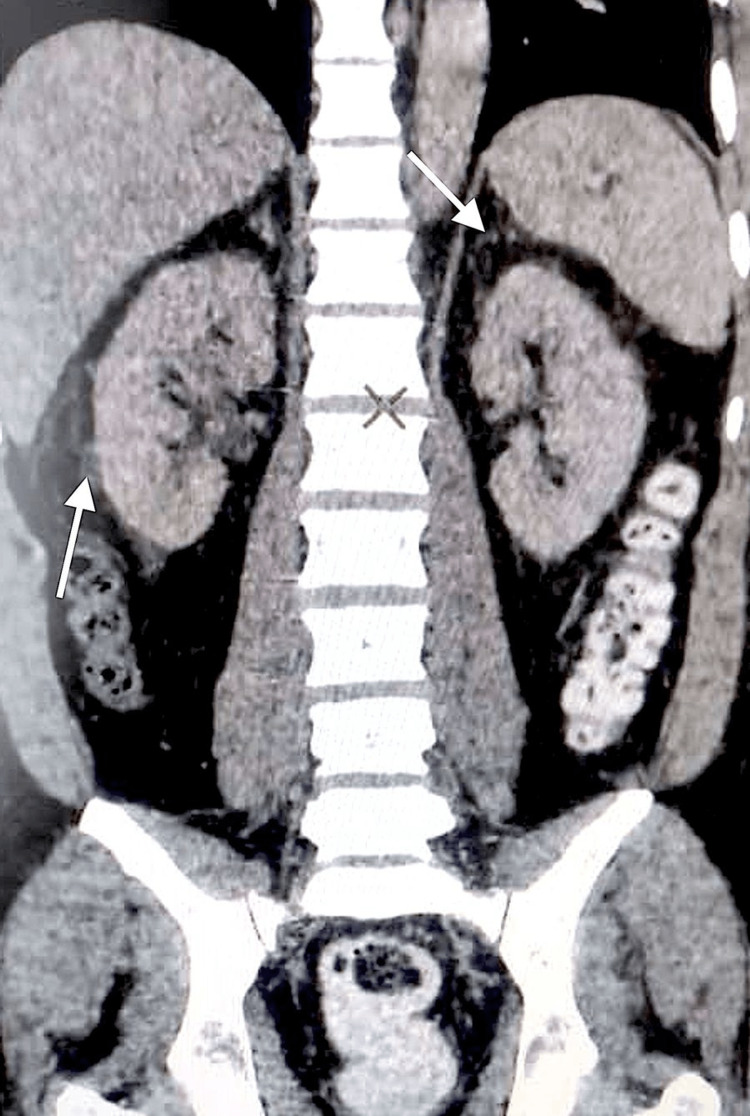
CT scan shows mild bilateral perinephric fat stranding (arrows).
